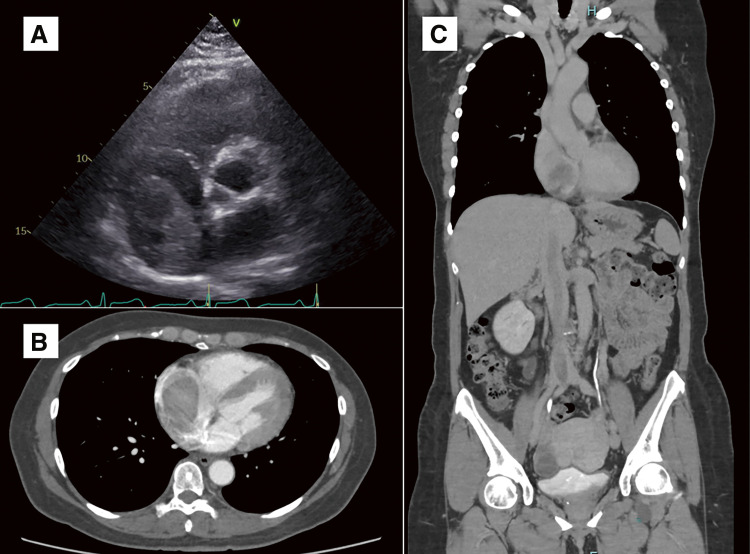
Fig. 1 (A) Preoperative TTE revealed a 6 × 4 cm moving structure within the right atrium. (B, C) Preoperative contrast-enhanced CT showed a 37-cm-long tumor extending from the right side of the uterine body through the right internal iliac vein and IVC to the right ventricle. TTE: Transthoracic echocardiography; IVC: inferior vena cava
